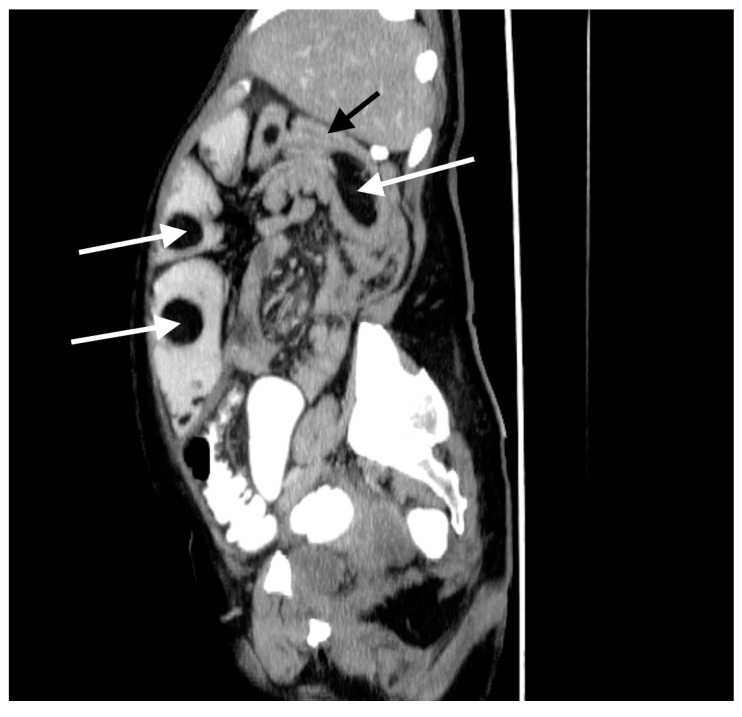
Contrast-enhanced CT image of the abdomen and pelvis, sagittal section. Multiple jejunal lipomas (white arrows) and intussuscepted jejunum with a lipoma as the leading point (black arrow).
